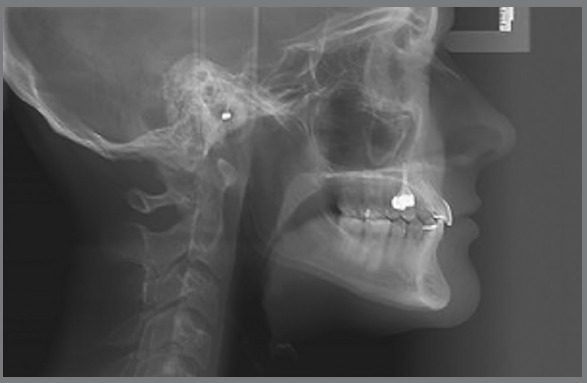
Lateral radiograph at the end of orthodontic treatment.
